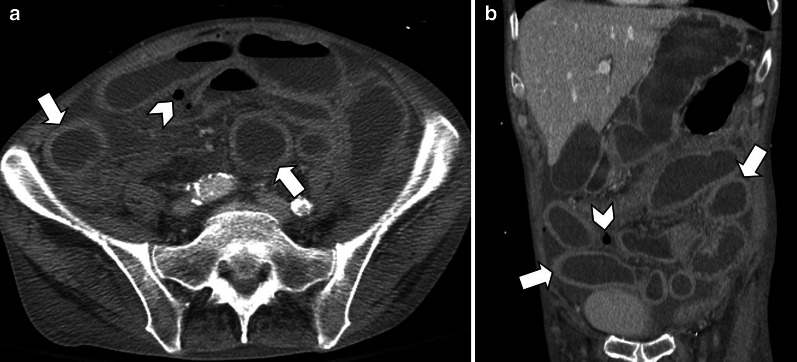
A 64 y/o male with remote history of abdominal liposarcoma, status post whole abdominal external beam radiation therapy. Diffuse marked small bowel wall thickening (thick arrows) is evident involving bowel loops within the radiation field associated with transmural enhancement suggestive of early ischemia. However, there is small volume pneumoperitoneum (arrowheads), due to CT-occult micro-perforation, suggestive of focal bowel necrosis
